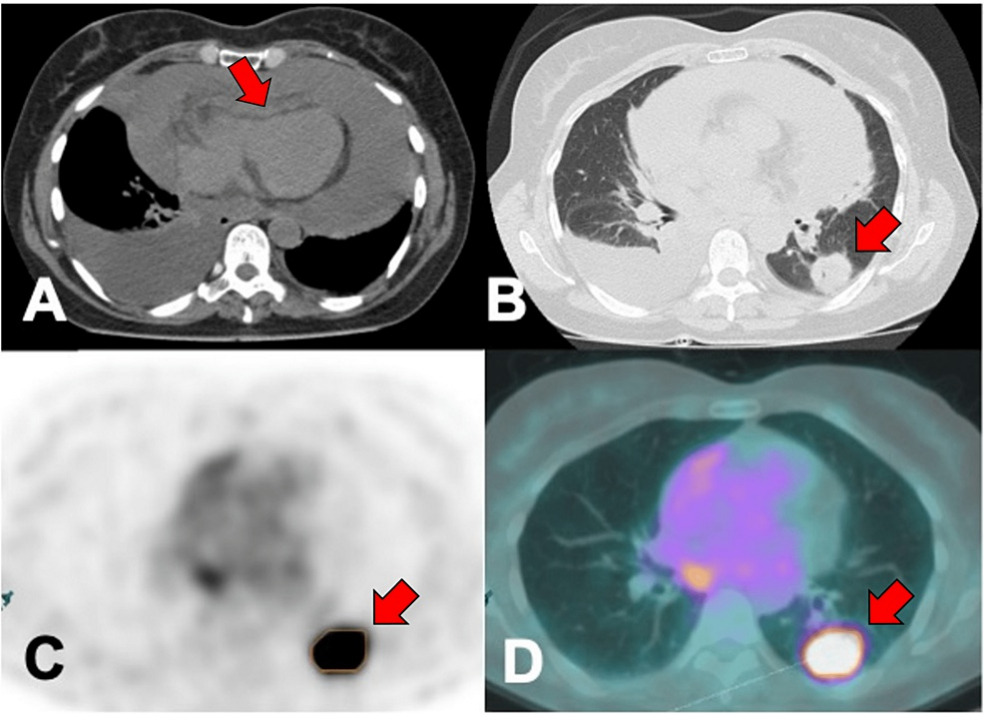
Imaging modalities in the evaluation of pericardial effusion. A: Chest CT scan showing a large-volume pericardial effusion with signs of right ventricular compression (arrow indicating the compressed right ventricle). B: Chest CT scan showing a suspicious lung lesion of nodular morphology with a necrotic center in the left lung (arrow). C and D: PET showing increased FDG uptake by the lung lesion (arrow). CT: computed tomography, PET: positron emission tomography, FDG: fluorodeoxyglucose
